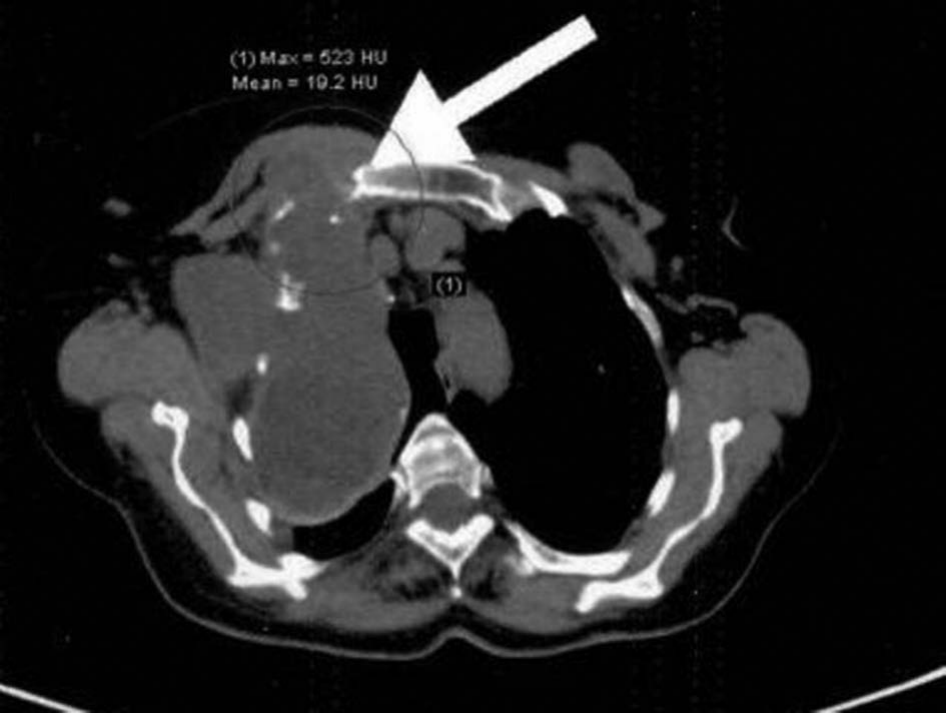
Preoperative Thoracic CT Image
